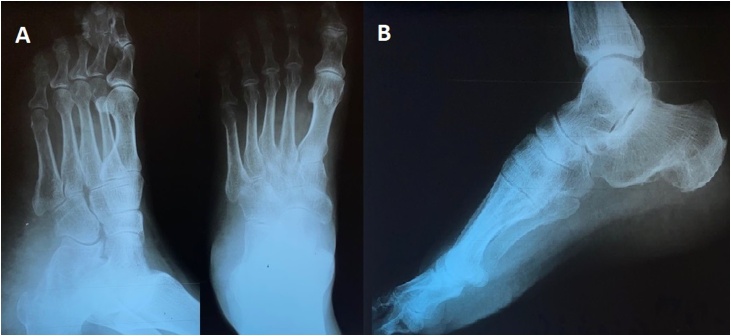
Plain radiograph: Anteroposterior views show soft tissue mass in plantar and heel of right foot (A), lateral view shows subcutaneous soft tissue mass in foot plantar and heel with slight calcaneus periosteal irregularity of calcaneus bone (B).
